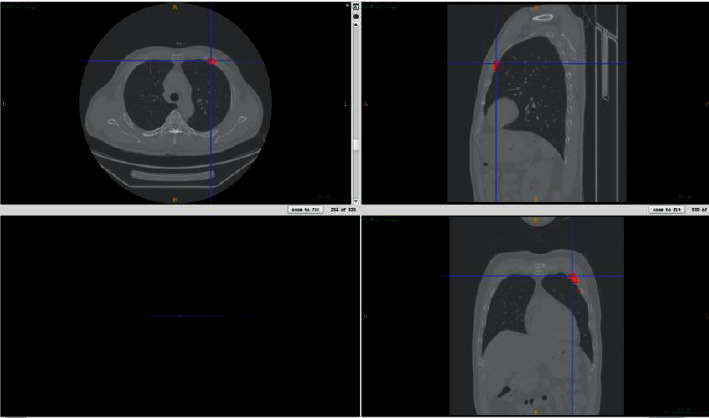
Example of a 3D rib CT image.
